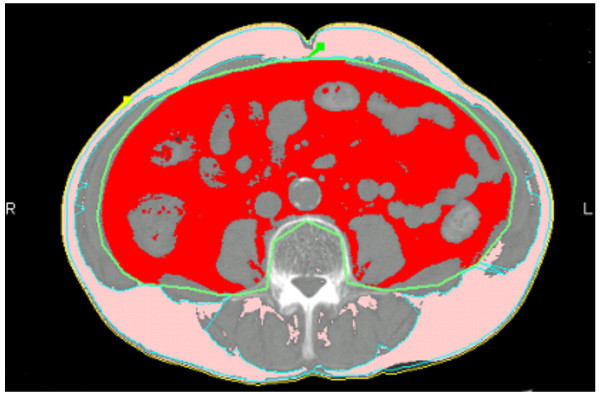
Distribution of abdominal fat as measured by FatScan software on a CT scan at the umbilicus level. The visceral fat area was regarded as red, and the subcutaneous fat area was regarded as pink.
